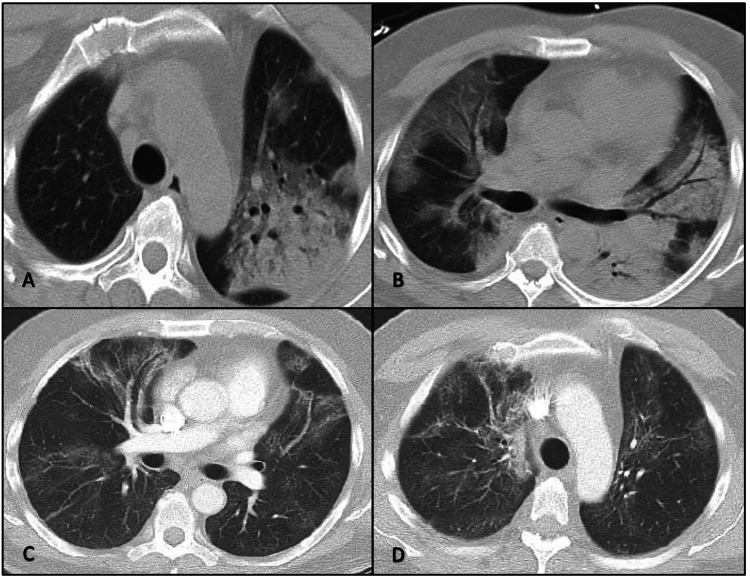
A and B: CT scan of the chest without contrast showing patchy and consolidative airspace opacities with air bronchograms and peripheral ground glass in the left upper lobe and bilateral lower lobes. C and D: CT scan of the chest with contrast showing improvement in opacities bilaterally after proper treatment with residual ground glass bilaterally.
